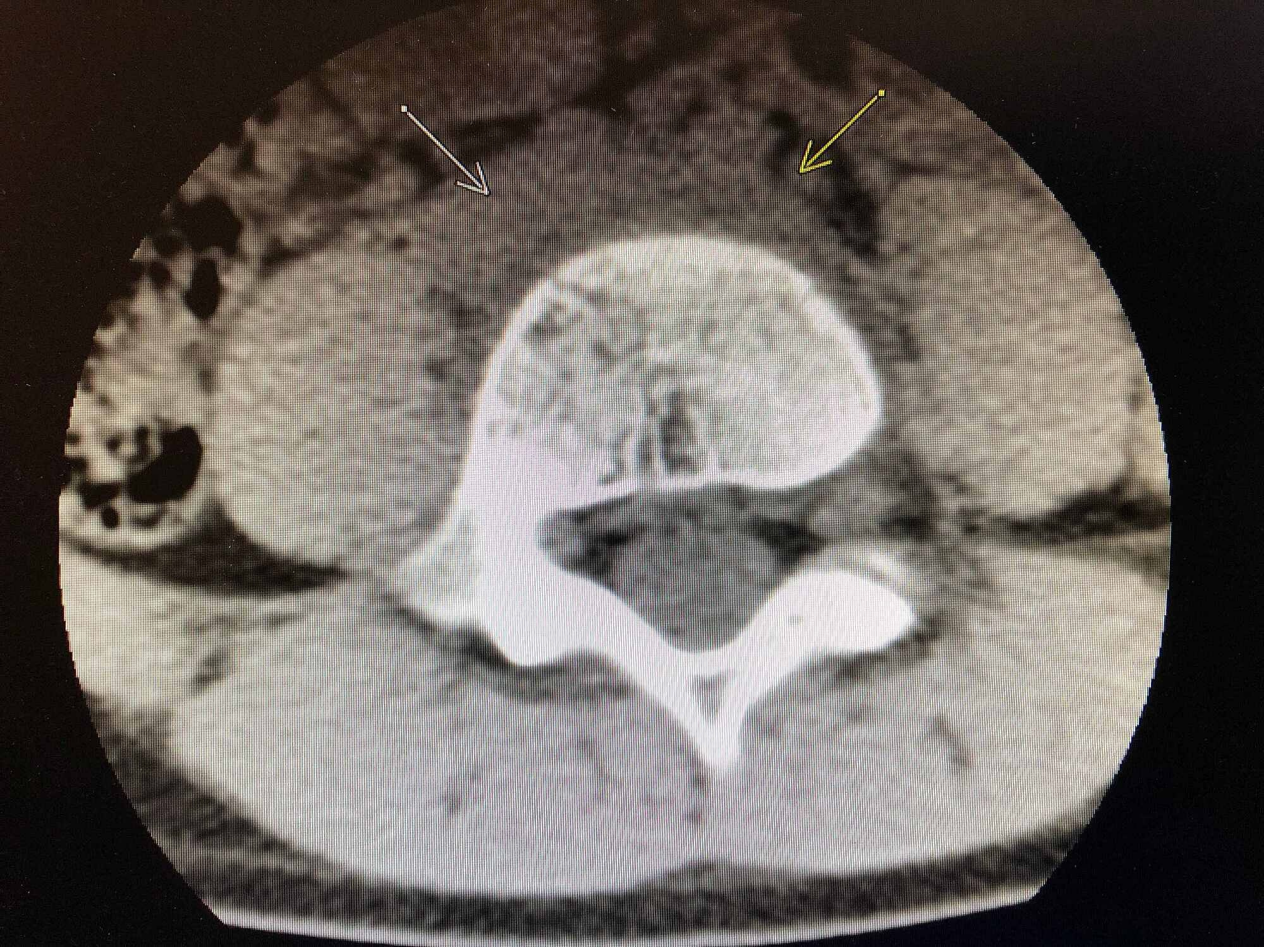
CT scan showing abnormal soft tissue thickening and edema anteriorly centered at the L4-L5 disc space level.
